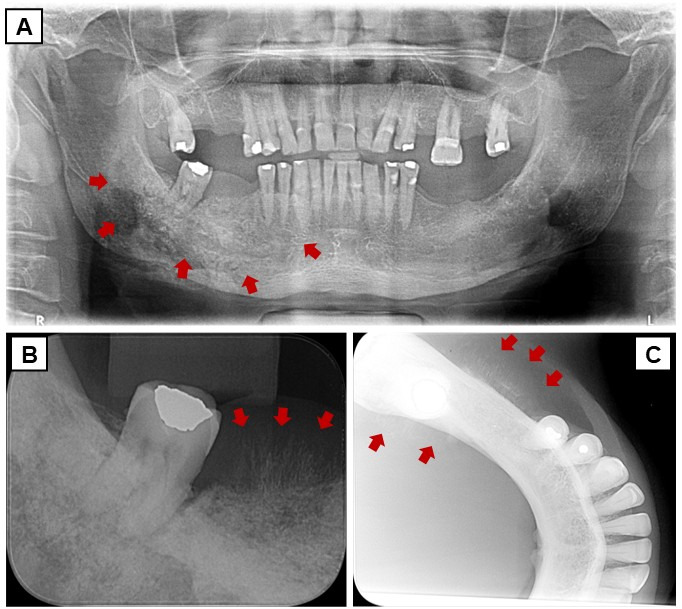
Radiographic characteristics of osteosarcomas of the jaws (panoramic, periapical, and occlusal radiographs). (A) A mixed radiolucent-radiopaque lesion with poorly defined margins affecting the right mandibular body and ramus. (B) Absence of the lamina dura and thickening of the periodontal ligament space around tooth 47. In the edentulous region, a periosteal reaction with a classic “sunburst” pattern is observed, characterized by radiopaque bone spicules arranged perpendicular to the cortical bone (red arrows). (C) Occlusal radiograph demonstrating the characteristic sunburst periosteal reaction shown in panel B (red arrows).
